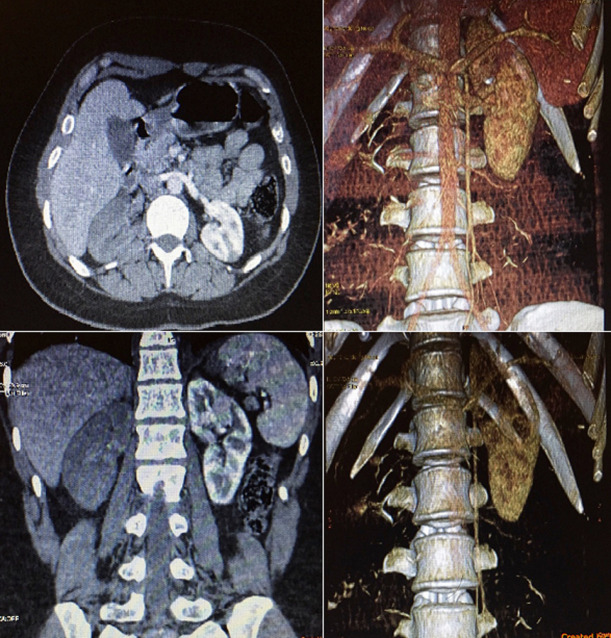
CT pelvic abdominal injected showing total renal infarction right and partial left confirmed at 3D volume-rendering-image
